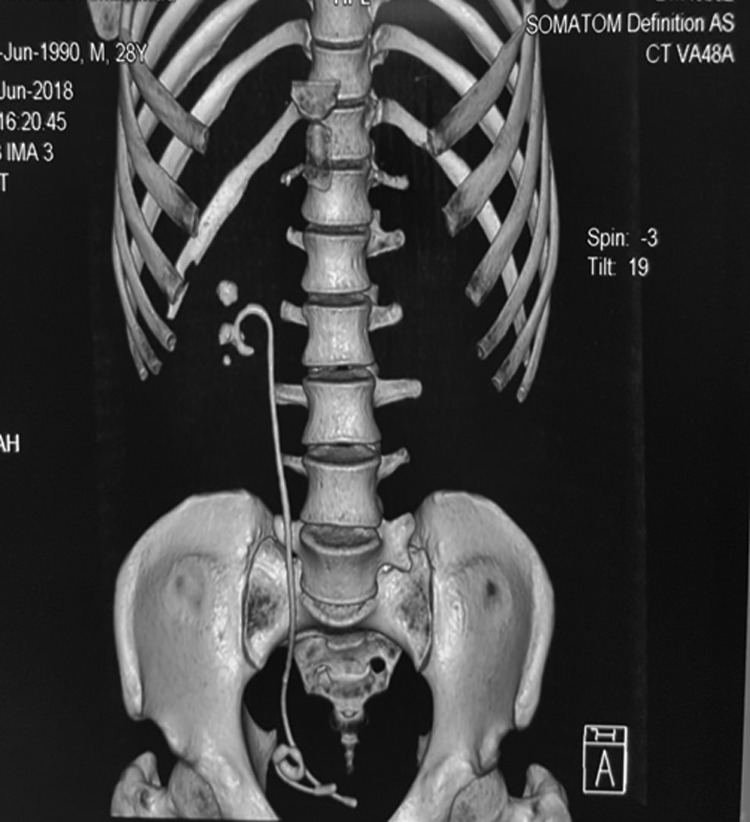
Native CT scan showed forgotten Double-J with a broken piece inside the urinary bladder.
